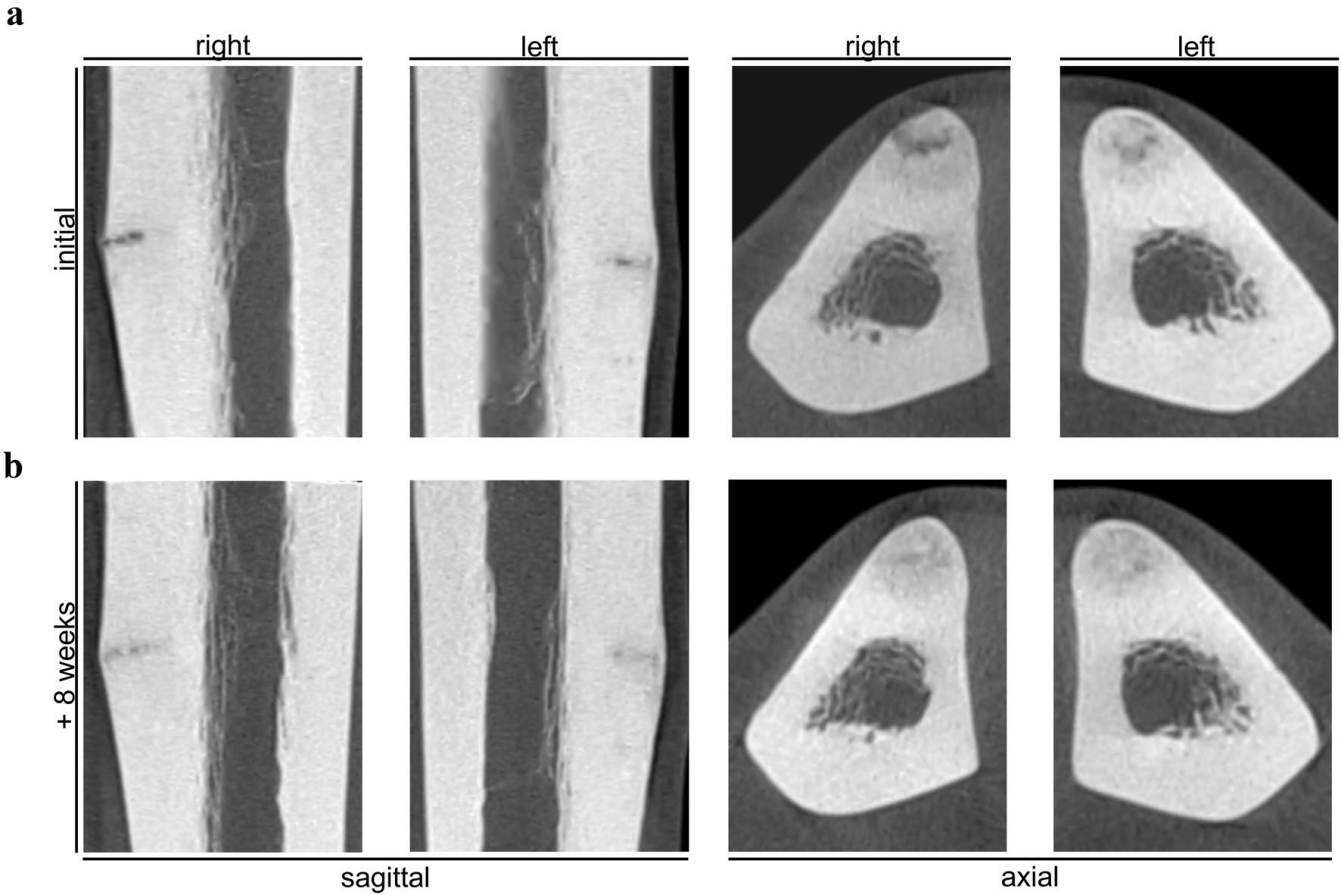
Course of lesion healing in Patient 4 assessed by cone beam computed tomography (CBCT). a Assessment of skeletal status at an initial presentation by CBCT revealed bilateral Looser zones at anteromedial tibiae. b Follow-up of radiograph after 8 weeks of intensified vitamin D supplementation (i.e., 14 days of 20,000 I.U. per day followed by 20,000 I.U. per week) and oral calcium gluconate supplementation (i.e., 1000 mg per day for three months) showed nearly complete consolidation in CBCT correlating to improved clinical symptoms
